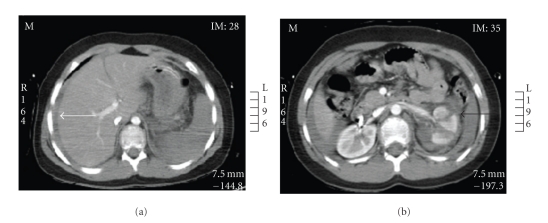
CT scan of the abdomen in portal venous phase showing fluid around the liver (white arrow), difficult to appreciate any splenic change. Lacerated left kidney with perinephric haematoma (black arrow).
